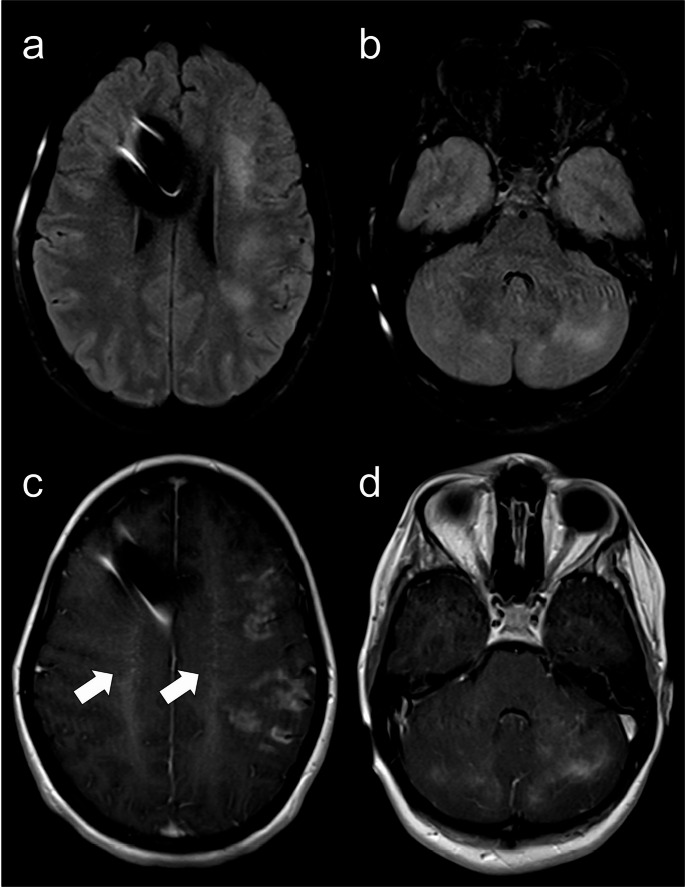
Hemophagocytic lymphohistiocytosis. A 23-year-old female patient with refractory septic shock in the context of hemophagocytic lymphohistiocytosis with predominant CNS manifestations. Genetic testing identified a homozygous variant with uncertain clinical significance in the UNC13D gene, associated with familial HLH. Brain MRI (a and b – axial FLAIR, and c and d – gadolinium-enhanced axial T1) demonstrates bilateral tumefactive, partly confluent, T2-hyperintense cortico-subcortical lesions with heterogeneous patchy enhancement, involving both supra and infratentorial regions. Prominent perivascular enhancement is noted on the centra semiovalia (arrows on c). A frontal ventriculoperitoneal shunt catheter is present
